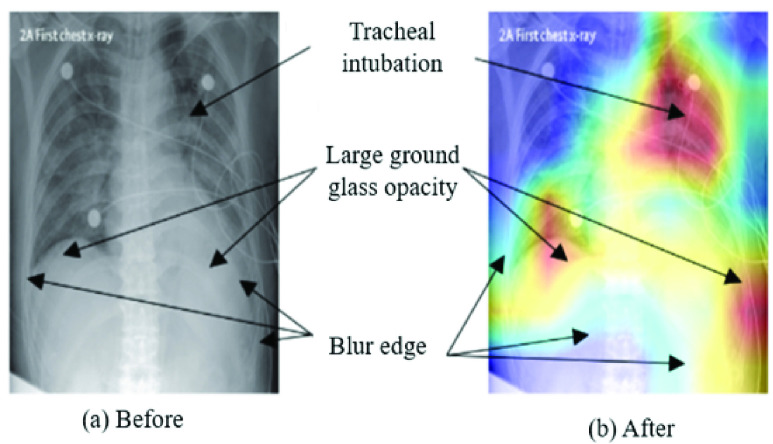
Model’s competency to identify essential features on chest X-ray using MobileNetV2.
